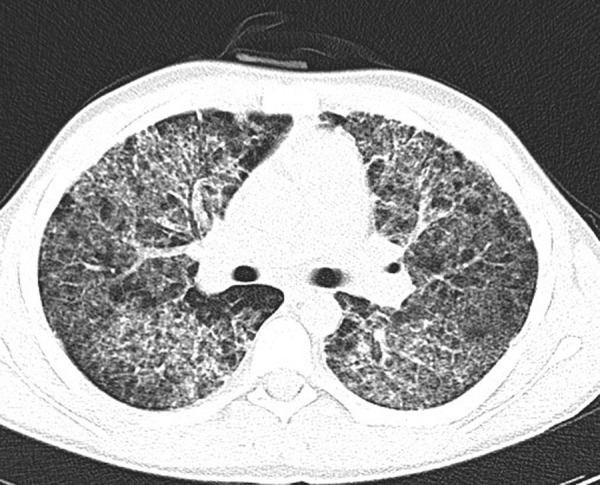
Chest CT. Bilateral ground glass opacities and diffuse centrilobular and peribronchovascular consolidation foci.
